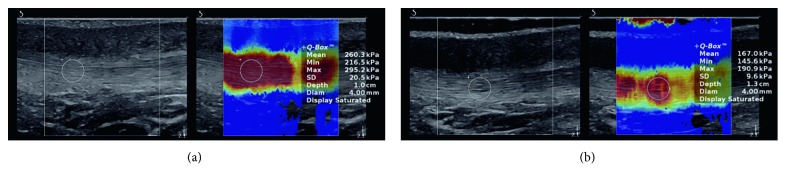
Quantitative evaluation of shear stress in the patella tendon using most saturated point of ultrasound images before (a) and after (b) the high-induction magnetic stimulation.
